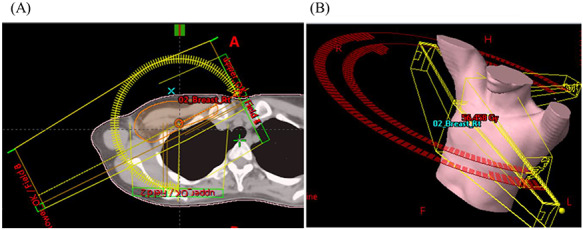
Beam arrangement of H-VMAT. (A) Axial CT image and (B) overhead view of beam setup. Hybrid VMAT (H-VMAT) combines conventional tangential field mainly for the chest area and VMAT mainly for the supraclavicular area and marginal zone.
